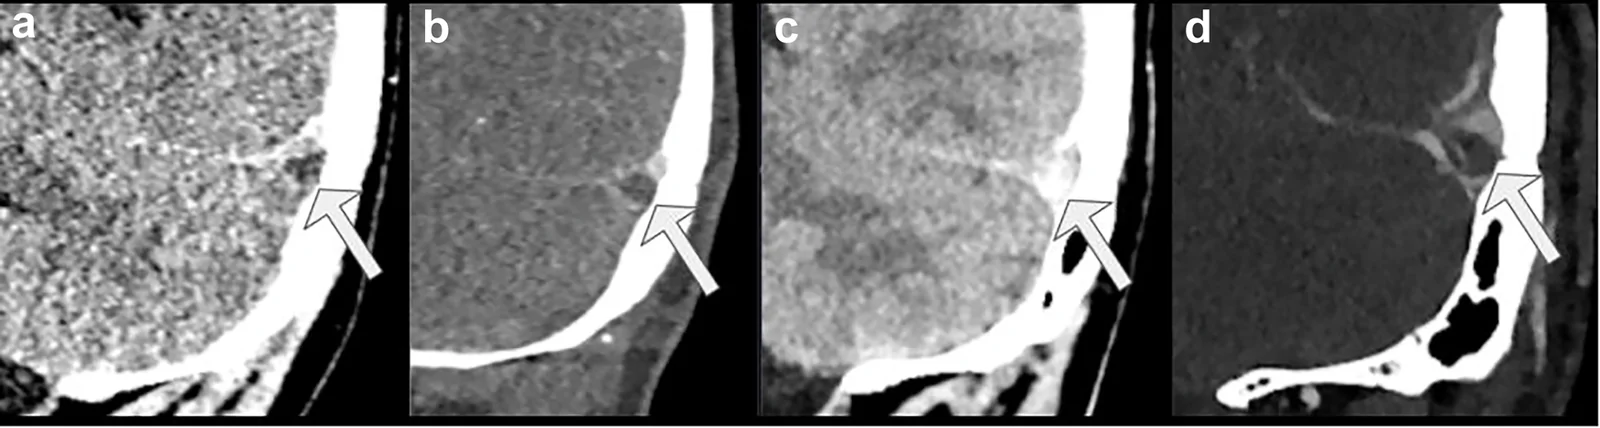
Evolution of blood-filled arachnoid granulation after subdural hemorrhage. a Non-enhanced CT shows normal CSF-attenuation arachnoid granulation in the left transverse sinus (arrow). b CT venography demonstrates a filling defect at the granulation site (arrow) with a tentorial subdural hematoma. c Follow-up non-enhanced CT shows hyperattenuating blood within the granulation (arrow). d Follow-up CT venography reveals a small vessel within the blood-filled granulation (arrow)
